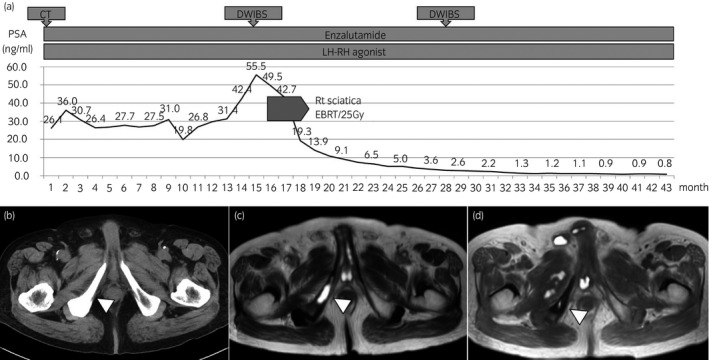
Case 1. A 74‐year‐old man with CRPC after RP and SRT (a). Enzalutamide administration is initiated. Sclerosis of right sciatica is detected with CT. (b) After the administration of enzalutamide, his PSA level initially remains at 20–30 ng/mL, but it increase within 1 year of enzalutamide treatment. Subsequently, DWIBS shows right sciatic metastasis (c). After 1 year of EBRT for right sciatic metastasis, the follow‐up DWIBS study shows intensity reduction in the lesion (d).
